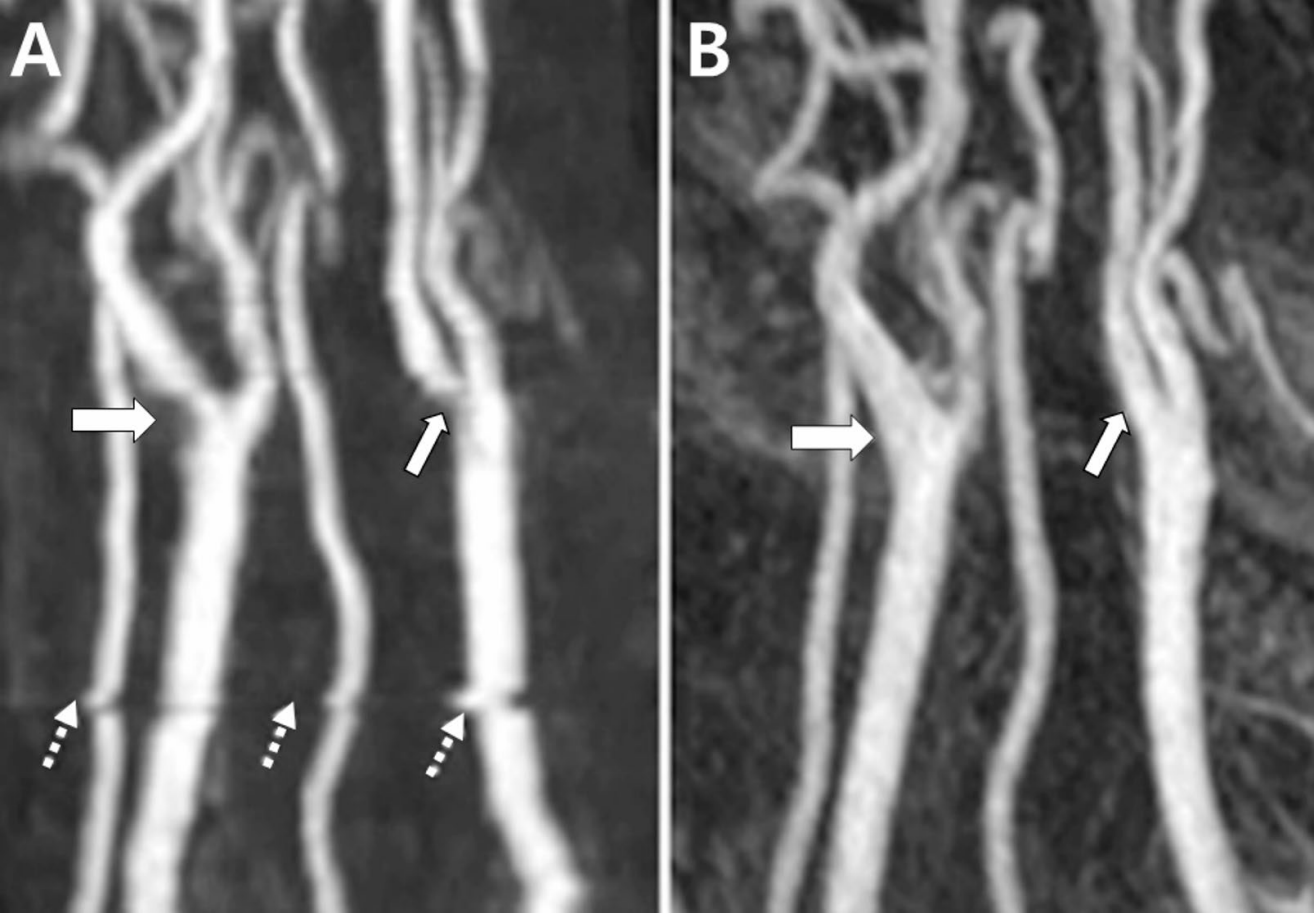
Stair-step artifacts. (A) Pseudo-stenosis caused by stair-step artifacts in 2D TOF MRA is observed in the right internal carotid artery (ICA) (thick arrow), left ICA (thin arrow), bilateral vertebral arteries, and left common carotid artery (dotted arrows). (B) In the contrast-enhanced MRA of the same patient, no artifacts are seen in the vessels indicated in (A). Figure adapted from McKinney AM: Artifacts of the Craniocervical Arterial System on MRI. In: *Atlas of Normal Imaging Variations of the Brain*,* Skull*,* and Craniocervical Vasculature*. Cham: Springer International Publishing; 2017: 1261–1291, under the terms of the Creative Commons Attribution License [16]
